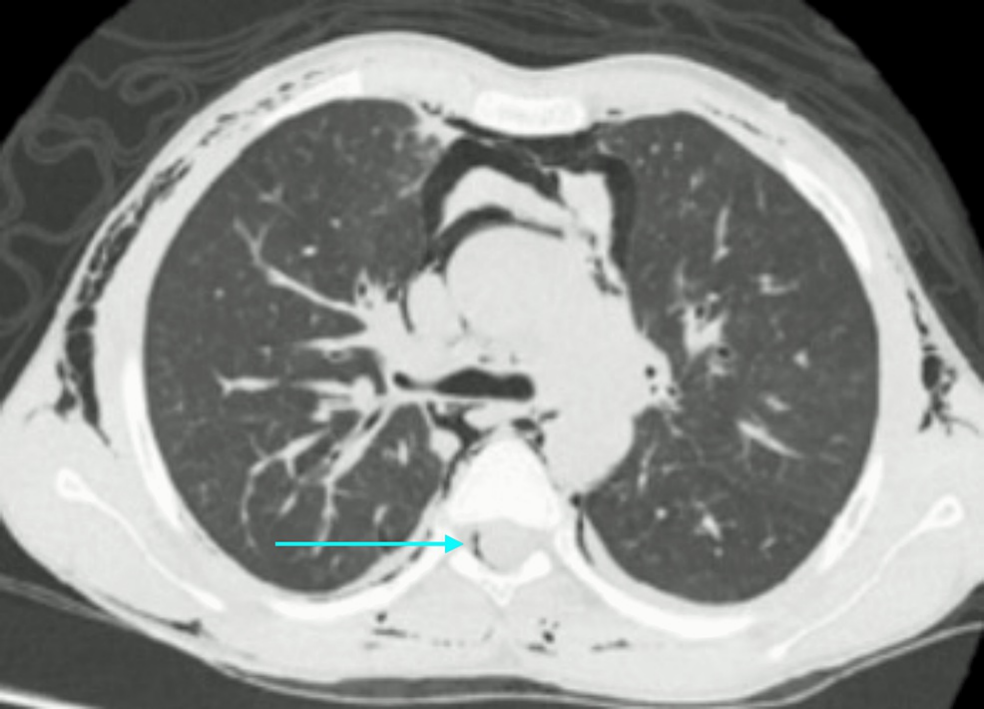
HRCT axial section through the level of carina Note the presence of epidural air (epidural pneumatosis)
